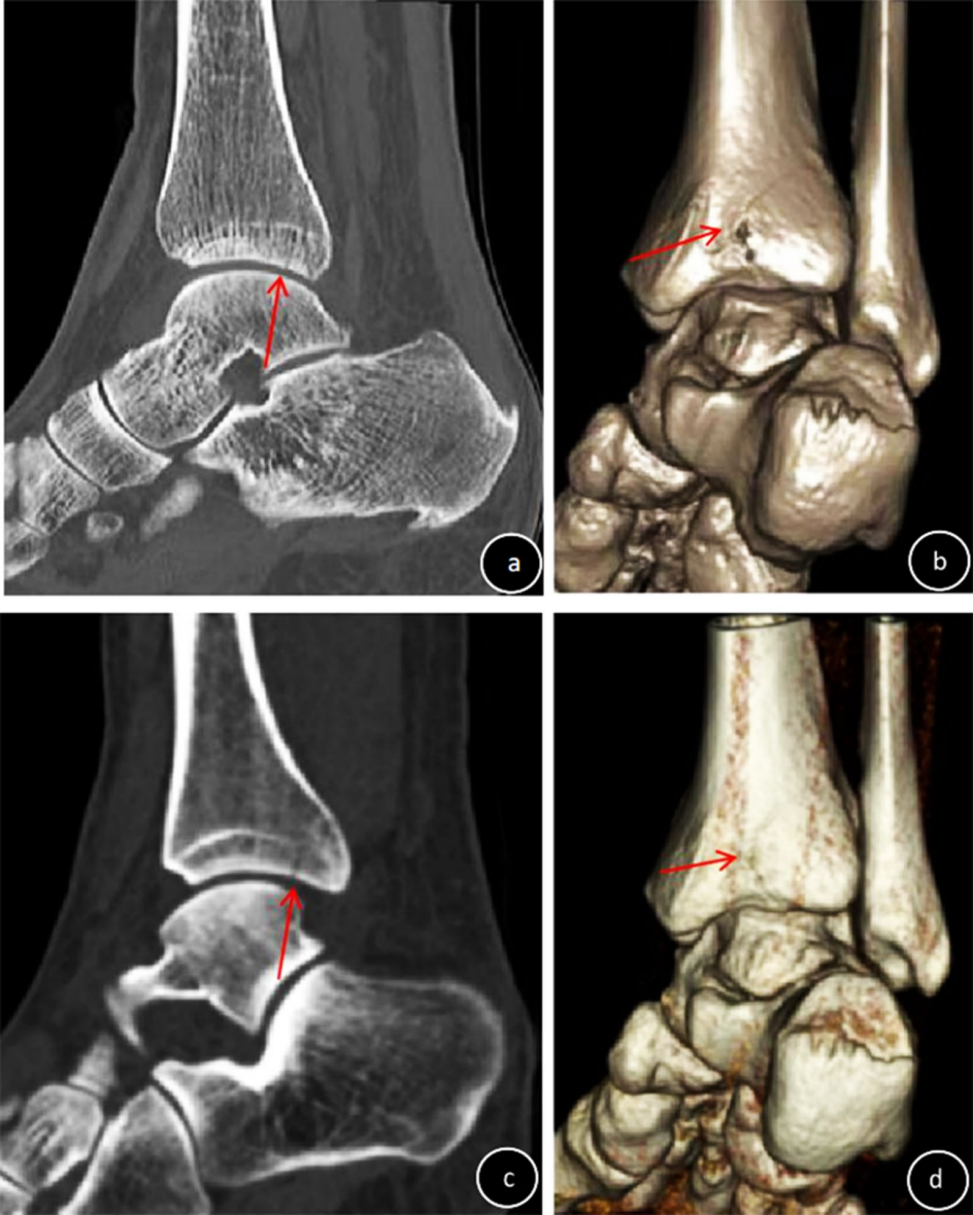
Missed diagnosis of non-displaced fracture of the ankle on SD-CT and ULD-CT (the fracture line can be seen as the red arrow). a SD-CT image (subjective CT image quality and impact of subjective CT image quality on clinical decision-making, 5 points); b SD-CT three-dimensional reconstruction; c ULD-CT image (subjective CT image quality, 4 points, impact of subjective CT image quality on clinical decision-making, 5 points); d ULD-CT three-dimensional reconstruction
